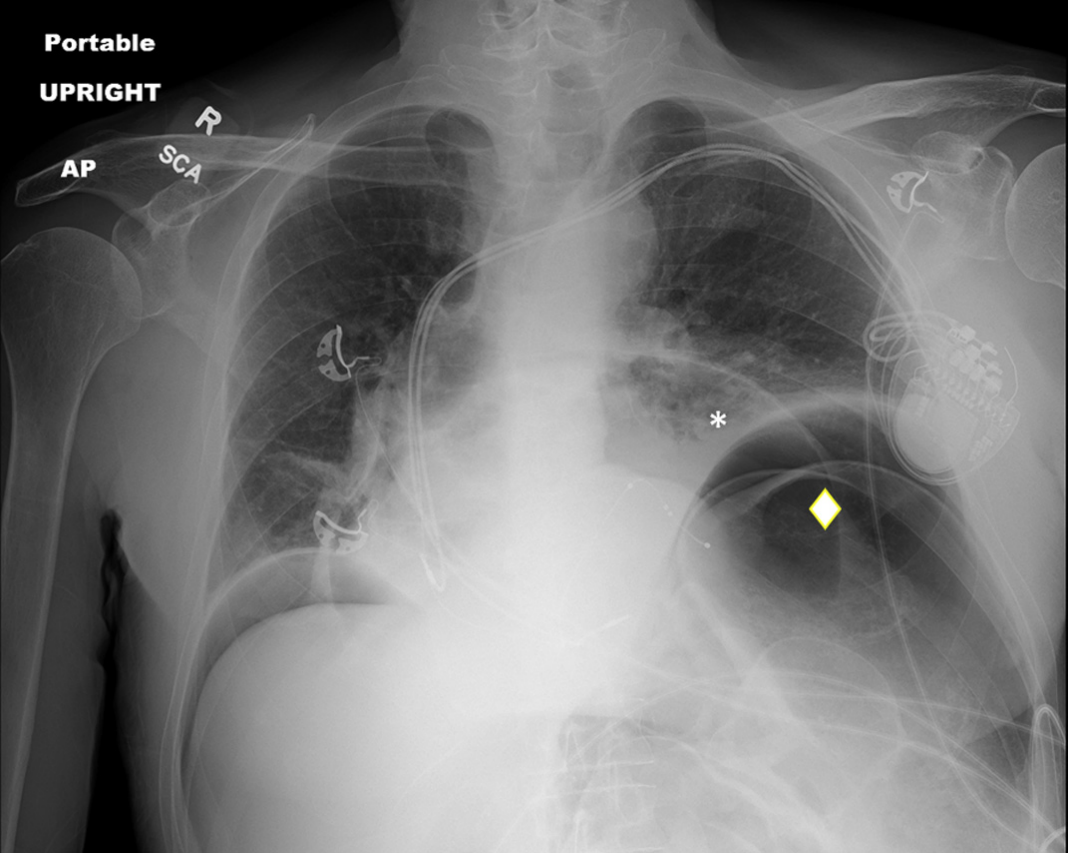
Chest Radiography Chest radiograph showing a large pneumopericardium (asterisk) and pneumoperitoneum (diamond). AP = anteroposterior; R = right; SCA = subclavian artery.
